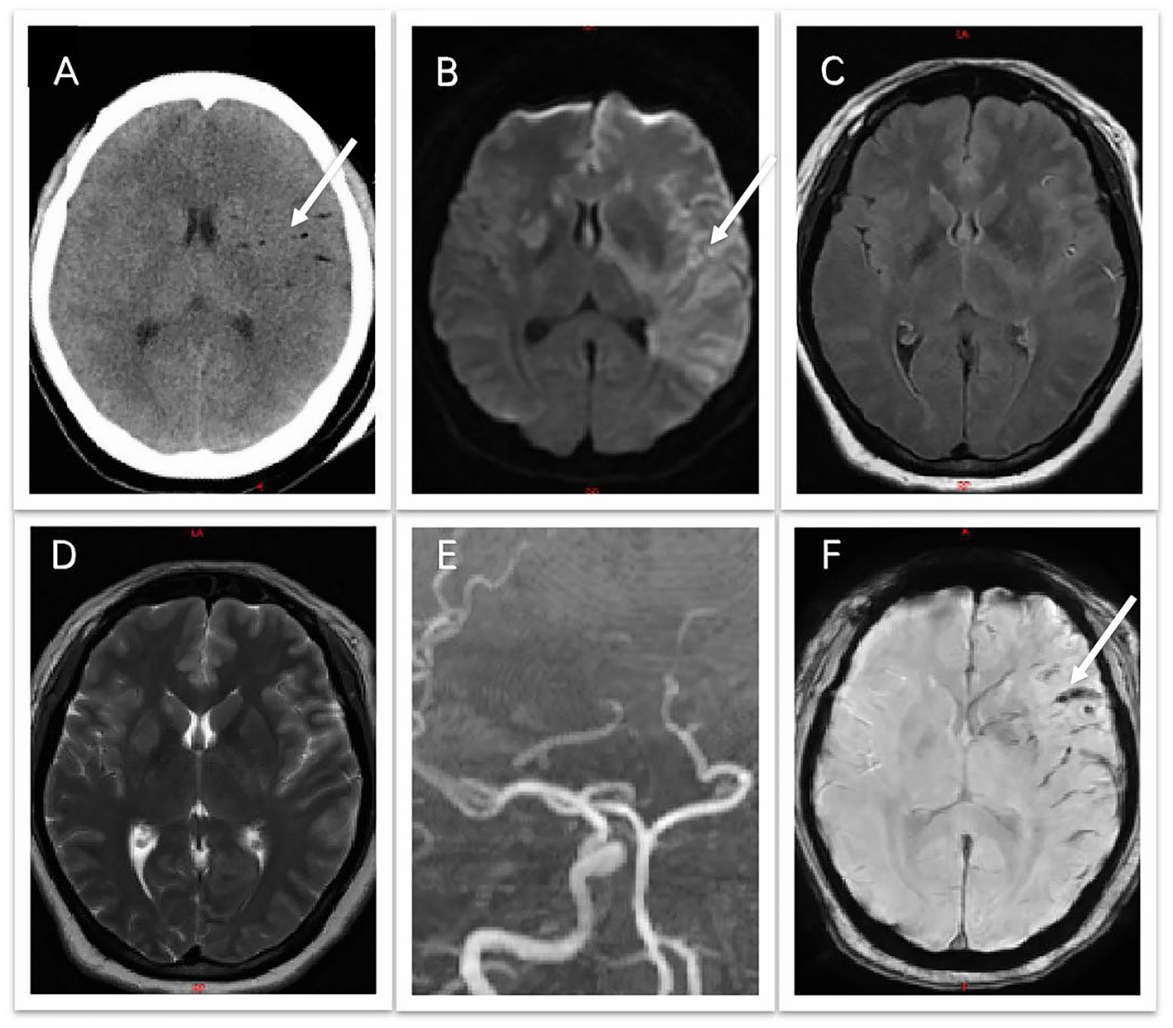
(A) Non-contrast computed tomography shows multiple low-density shadows along the blood vessels. (B) Diffusion-weighted imaging shows high-signal intensities in the left cerebral hemisphere. (C) Fluid attenuated inversion recovery. (D) T2-weighted sequences show much smaller lesions. (E) Magnetic resonance angiography shows occlusion of the left internal carotid artery, anterior cerebral artery, and middle cerebral artery. (F) Susceptibility-weighted imaging shows hypointensity along the cerebral vessels, indicating slowed blood flow caused by the fat emboli.
